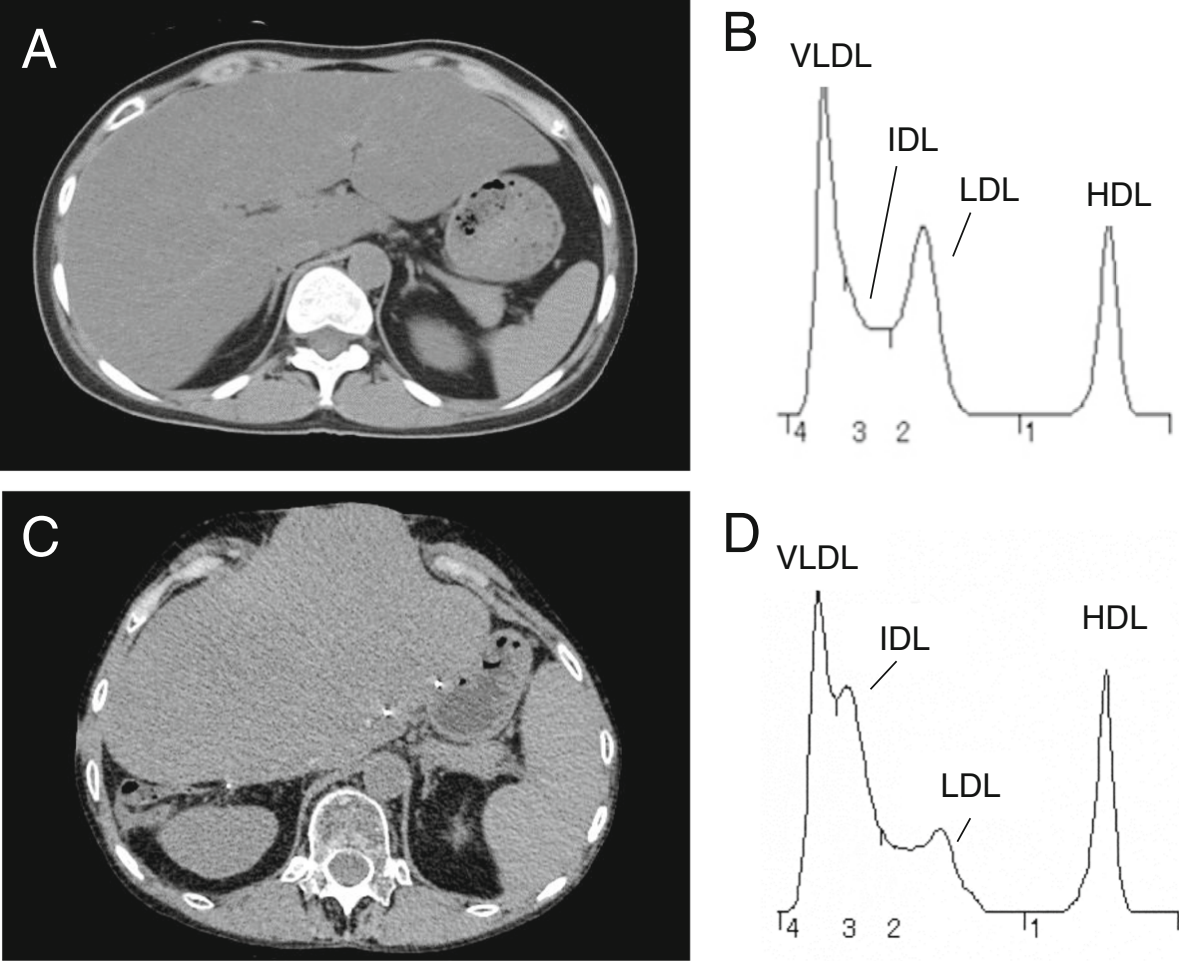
Computed tomography and polyacrylamide gel electrophoresis of two cases with lipoatrophy. a and c. Abdominal computed tomography of Case 1 (a) and Case 2 (c). Prominent fatty liver is observed. b and d. Acrylamide gel electrophoresis of Case 1 (b) and Case 2 (d). The elevation of VLDL and IDL is notable. *IDL* intermediate-density lipoprotein, *LDL* low-density lipoprotein, *VLDL* very low-density lipoprotein
